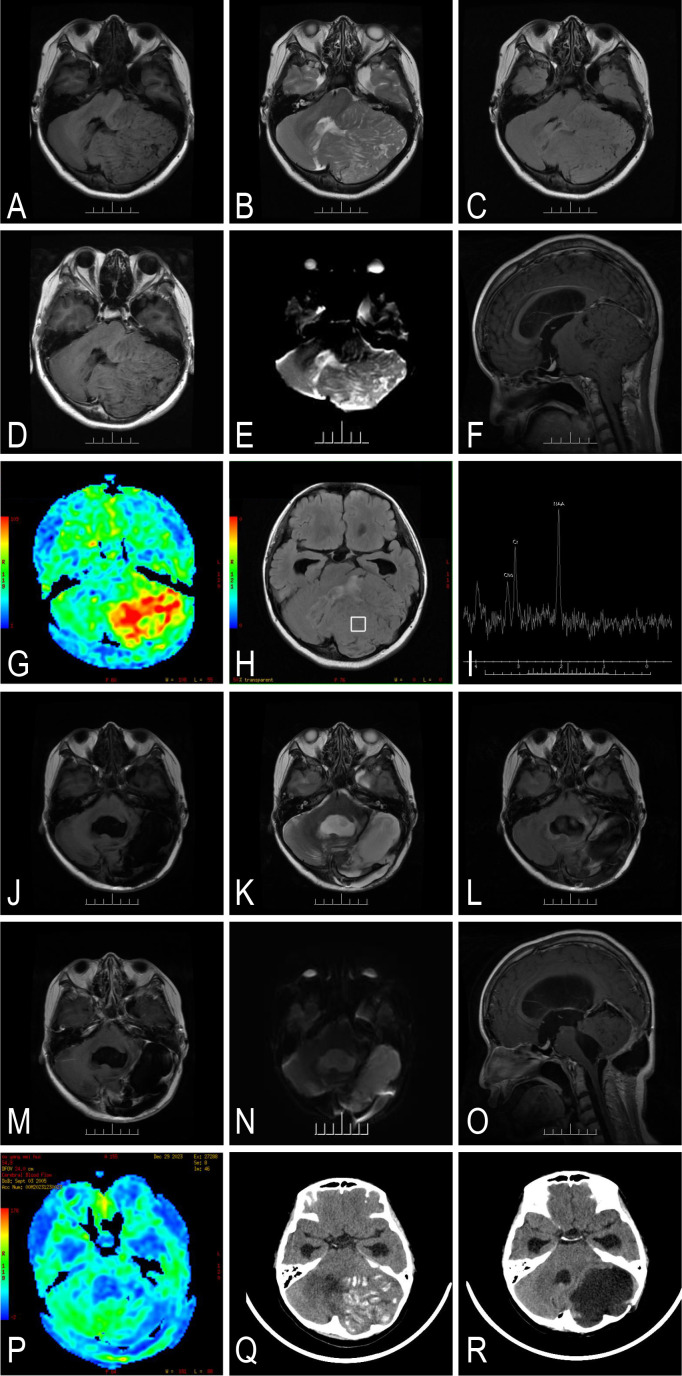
Representative imaging of case 3 in the perioperative period. It showed a heterogeneous lesion with T1 hypointense (A) and T2 hyperintense (B) abnormal signals in the left cerebellum, brainstem, and spinal canal. A slight edema and “tiger-striped appearance” in T2- and fluid-attenuated inversion recovery (FLAIR)-weighted imaging (C), heterogeneous linear contrast enhancement in T1-weighted imaging (D), and non-diffusion restriction in DWI are shown (E). The fourth ventricle is displaced, the cerebellar tonsil is compressed and deformed, and the tumor grew into the spinal canal at the C2 level (F). ASL imaging presents hyper-transfusion in the target area, suggesting a rich blood supply (G). The results of MRSI exclude glioma and medulloblastoma (H, I). The lesion shows multiple heterogeneous strip-like calcifications in CT (Q). The patient obtained STR, and part of the tumor remains on the side of the brainstem and below the pineal gland for preservation of neurological function (J–P, R).
